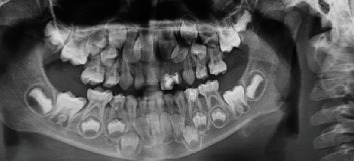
Panoramic radiograph showing the morphology of tooth 22 with a slight developmental delay and rotation.
